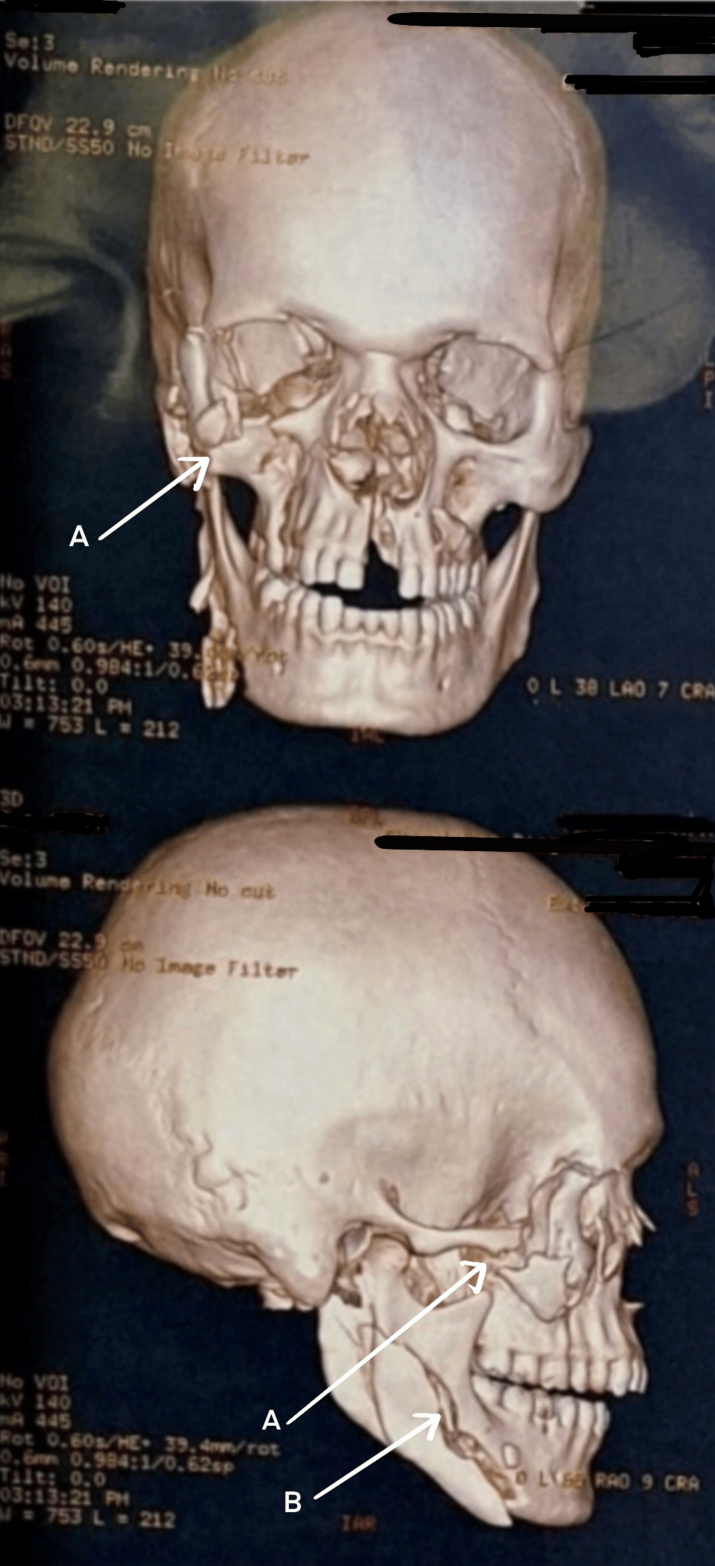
3D CT of the face and skull (Case 4) (A) Fracture of the right zygomatic complex with palatal split. (B) Fracture of the right ramus of the mandible.
